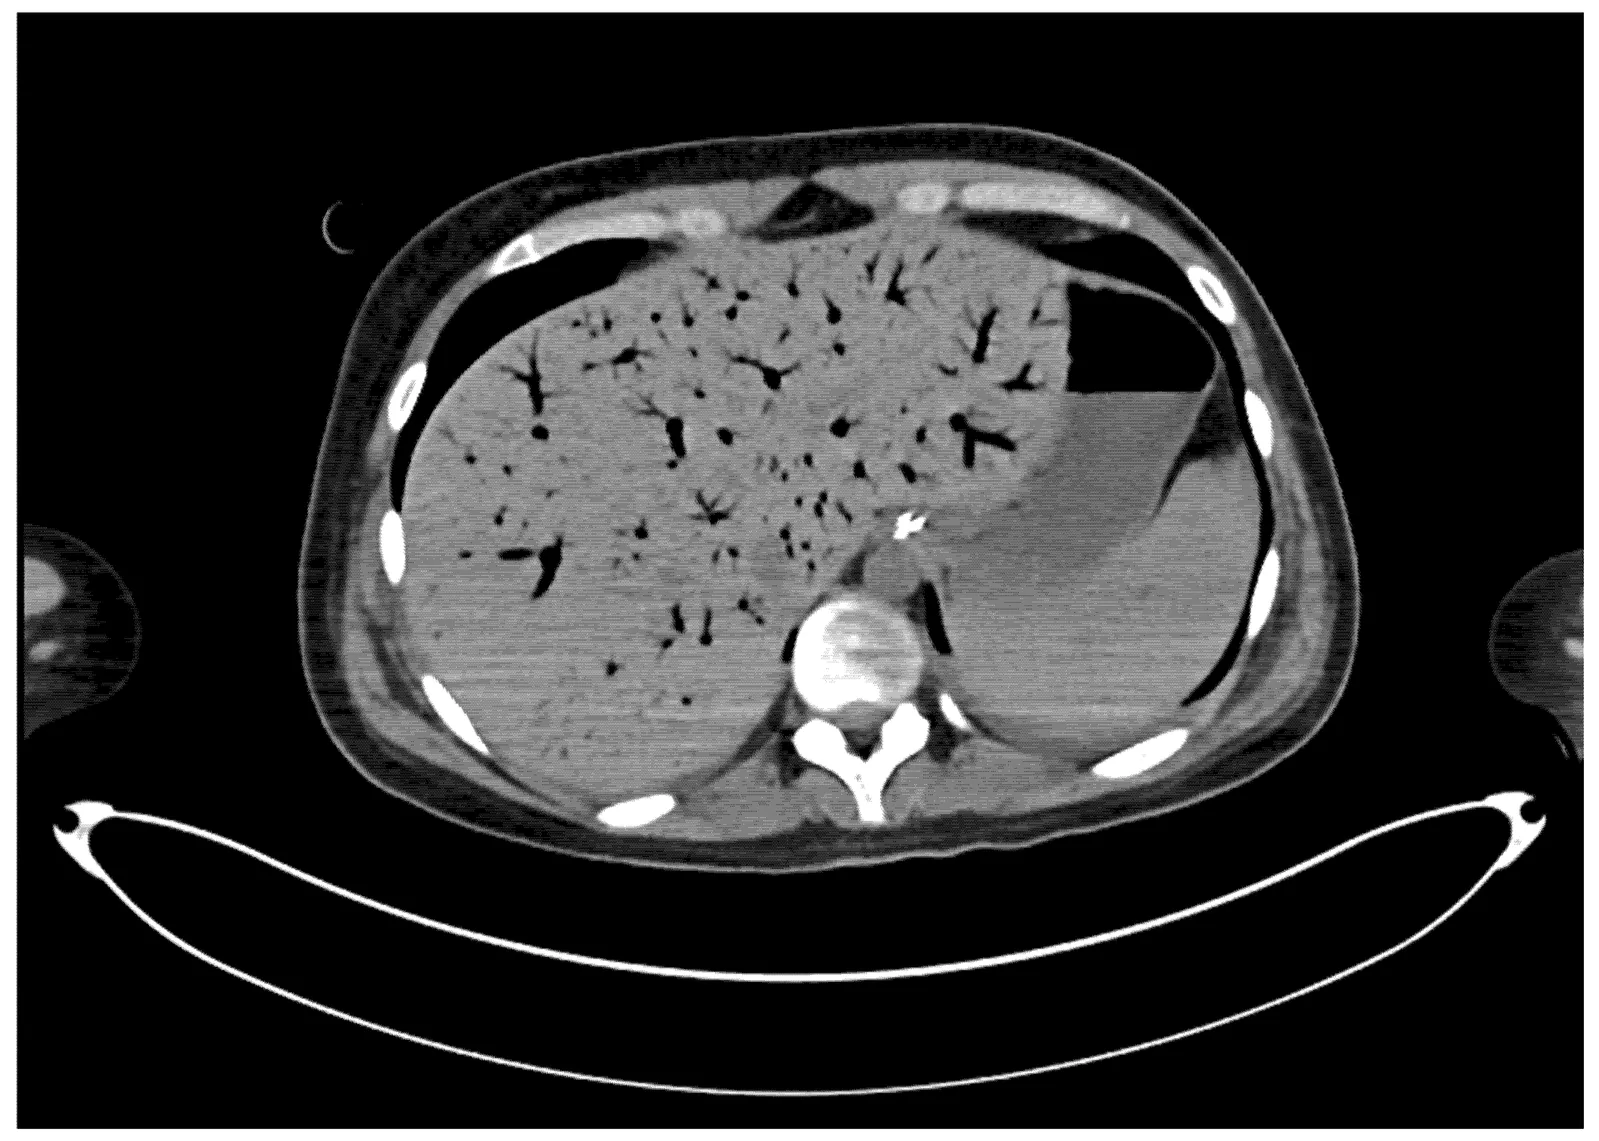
Abdominal CT imaging demonstrating extensive portal venous gas in the acute phase of toxic epidermal necrolysis.
